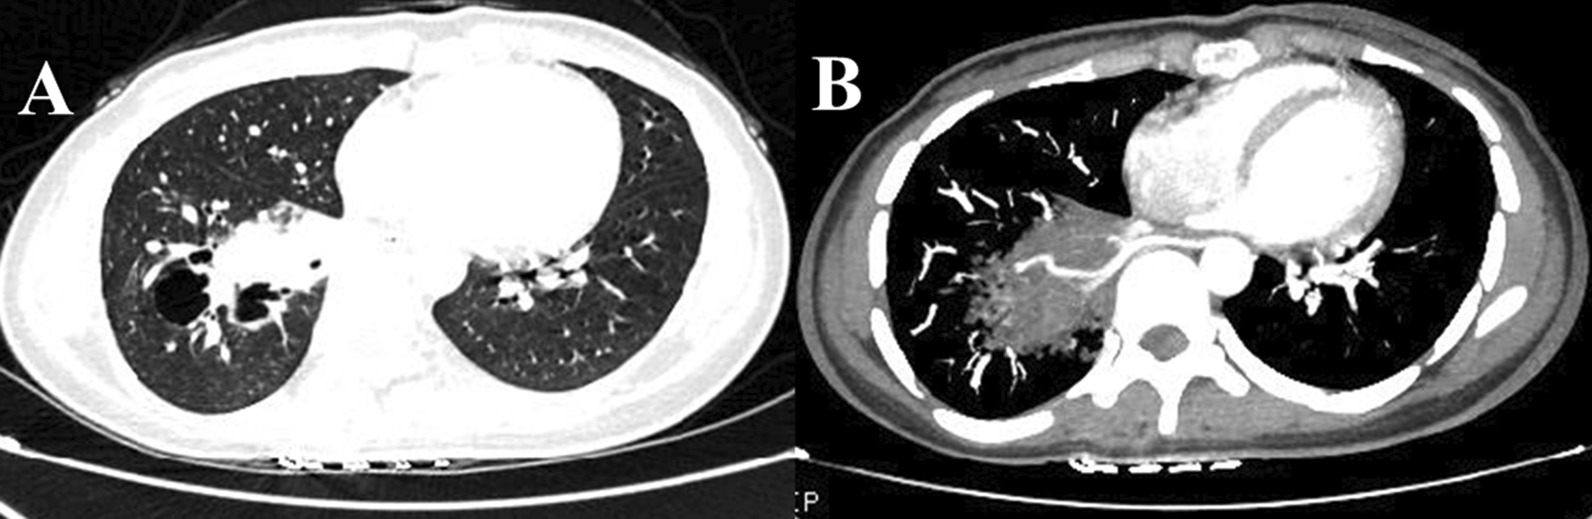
The sequestrated lung located in the right lower lobe was diagnosed using conventional two-dimensional computed tomography. A The lesion mimics bronchiectasis and infection; B contrast-enhanced images revealed the aberrant feeding vessel
